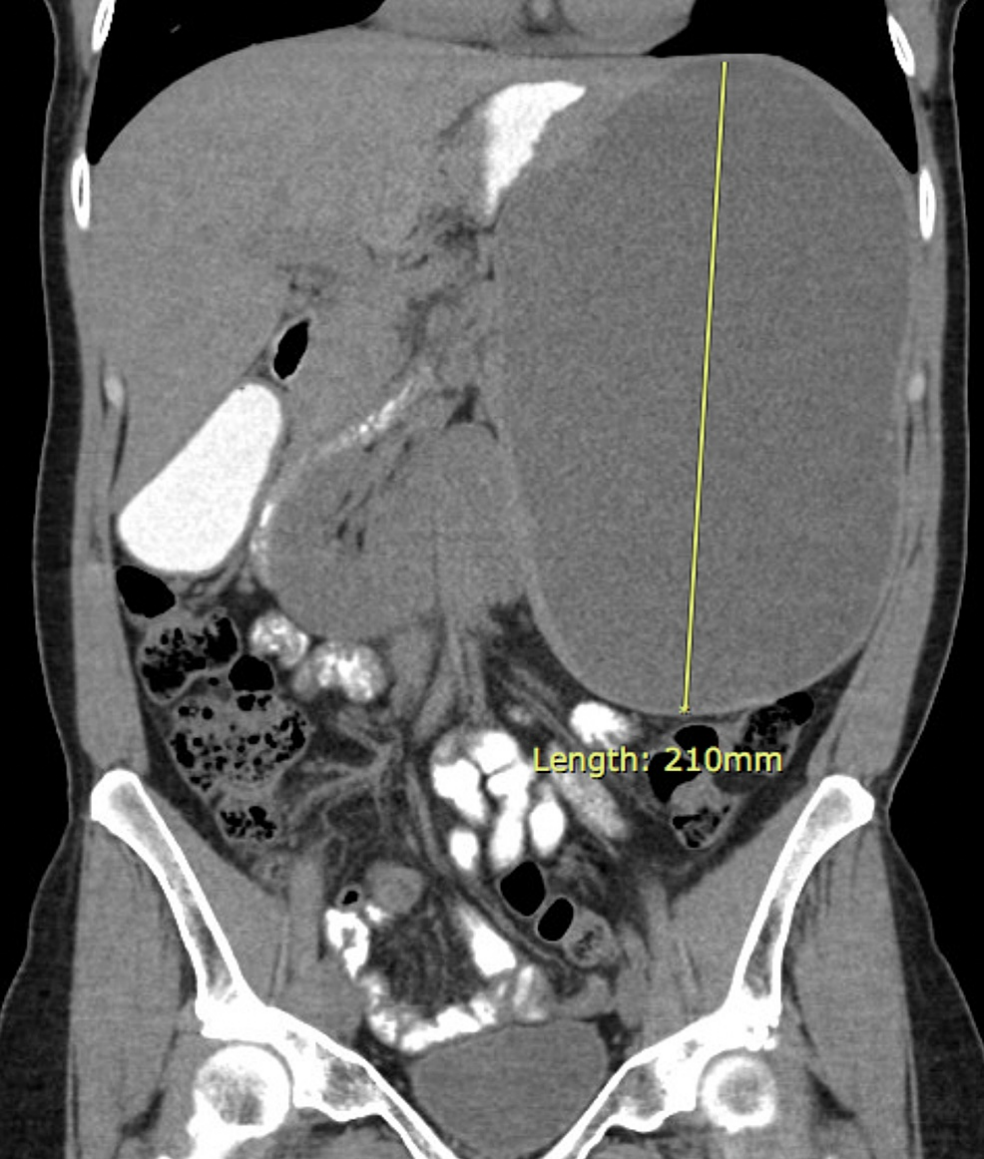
Abdominal CT scan demonstrating the splenic cyst
